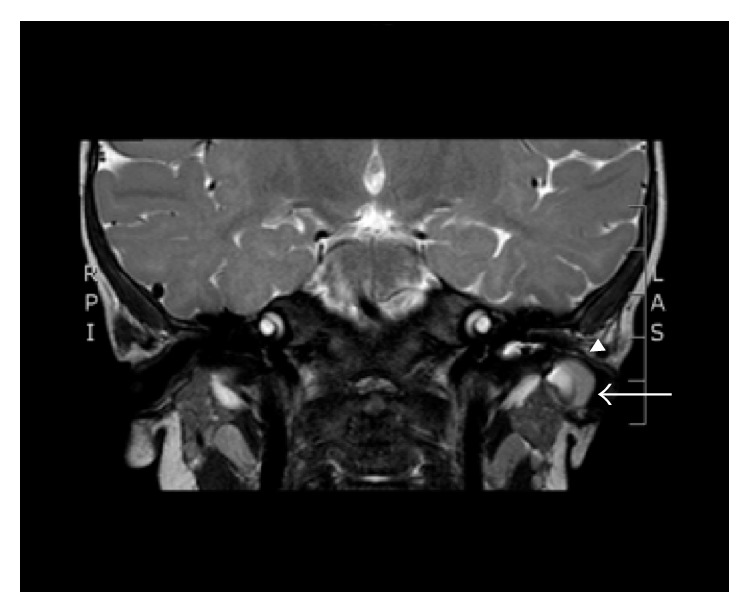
Case  1. MRI, coronal cut in T2 sequence, showing location of mass lateral to left parotid (arrow) and exerting mass effect towards the left EAC (arrowhead).
